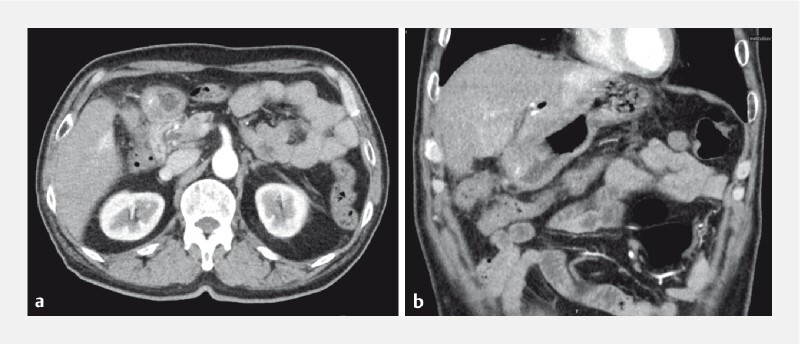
Computed tomography images of a gastric antrum submucosal lesion.
